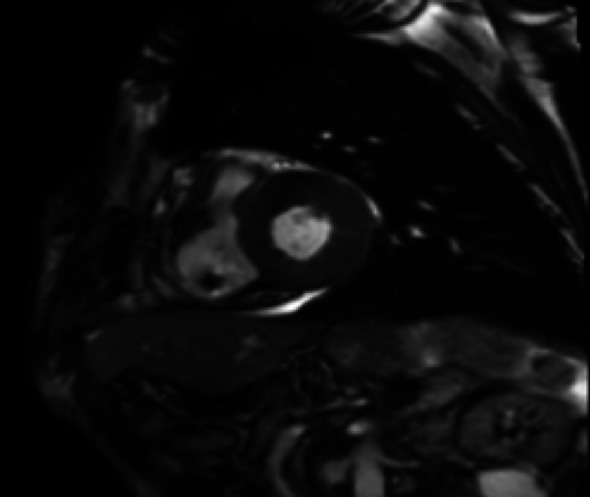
Short-axis T2-weighted image showing thickened myocardium without edema
